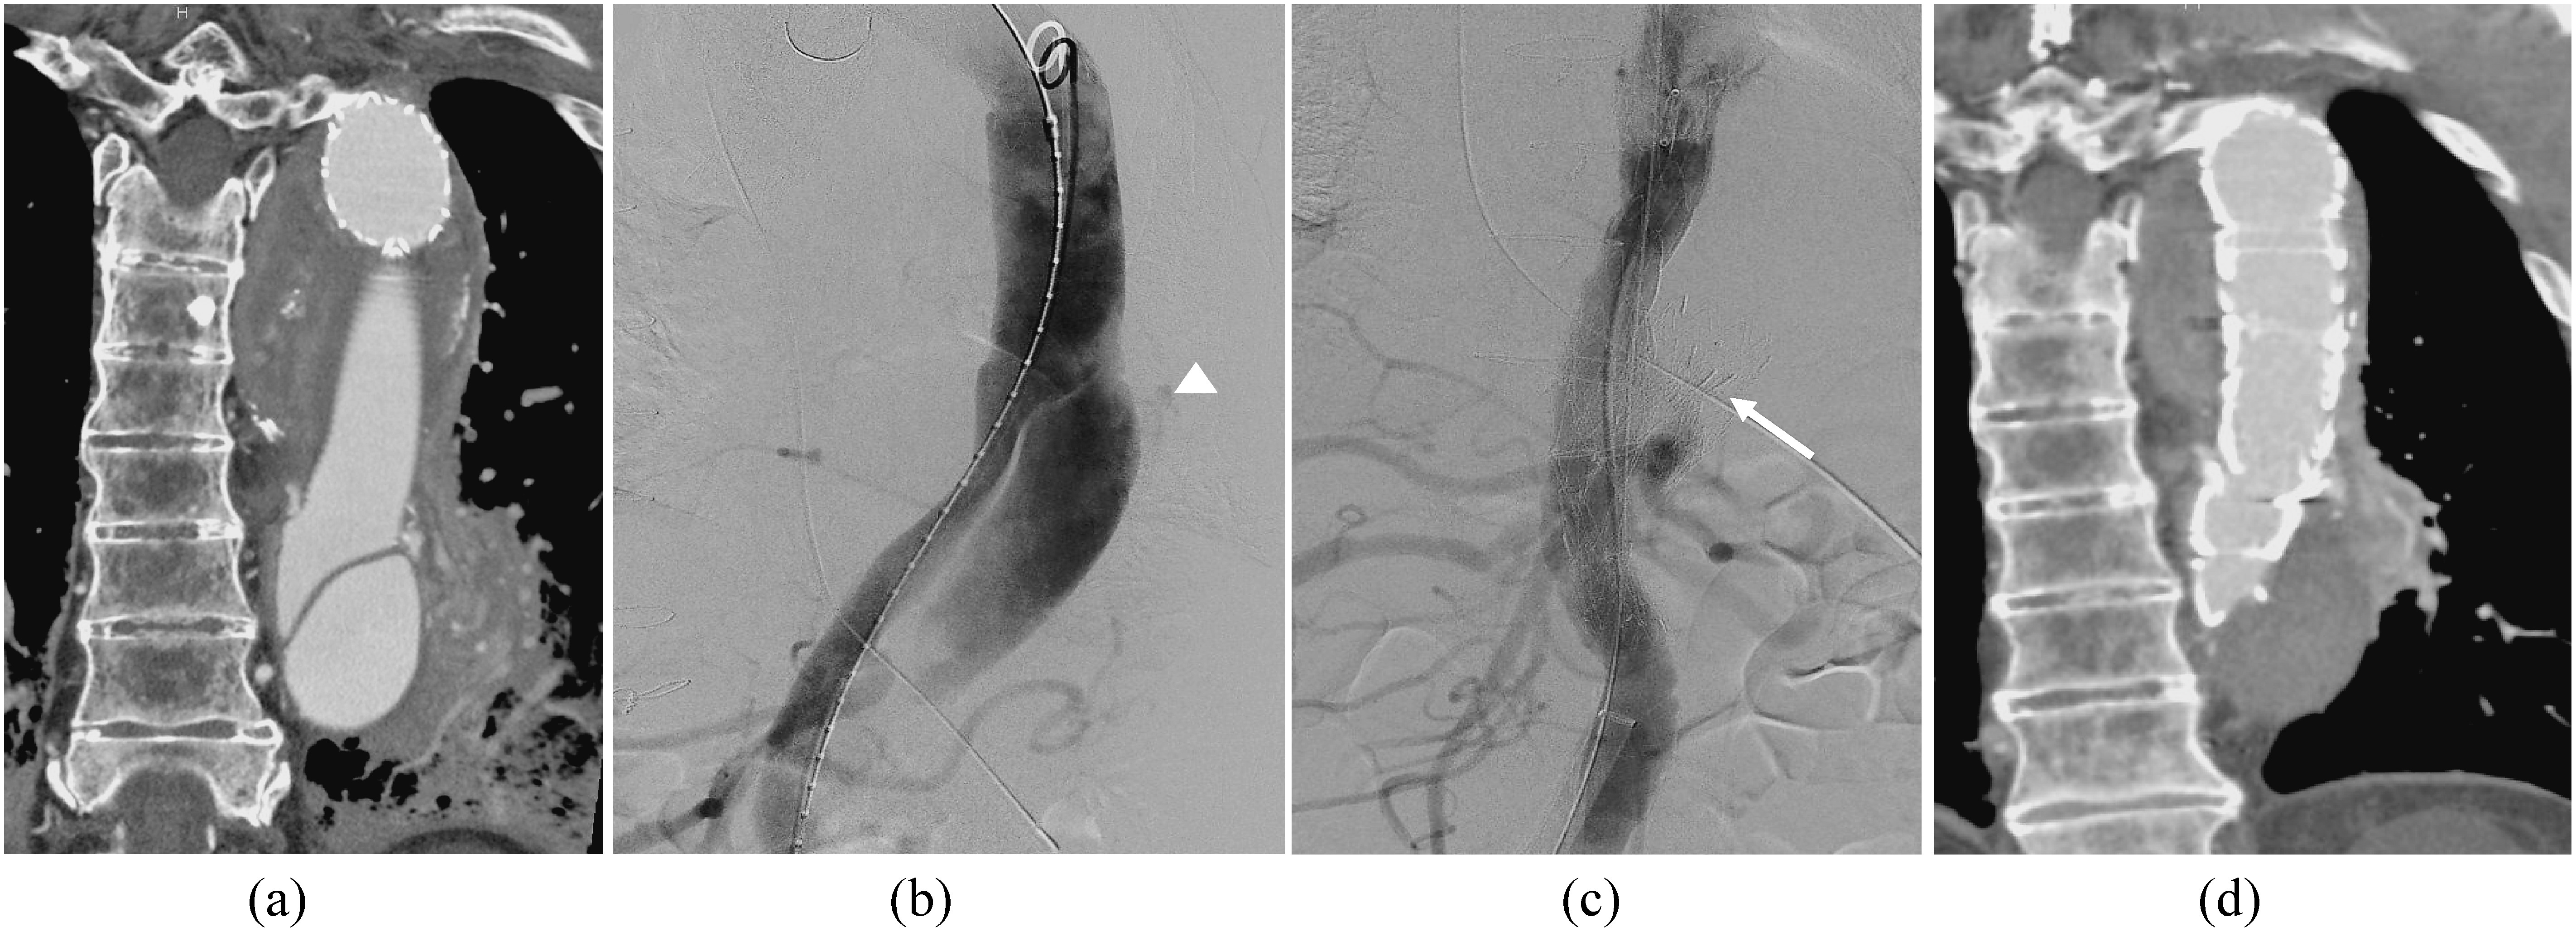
Fig. 6 A man in his 70s with hemoptysis after type A aortic dissection treated by total arch and descending aorta replacement and TEVAR. (a) Preoperative CECT shows hematoma around the anastomosis. (b) Aortography shows minor extravasation (arrowhead) close to the anastomosis and multiple distal entry points. (c) Postoperative TEVAR with the candy-plug technique shows no residual FL flow above the candy plug (arrow) on completion aortography. (d) Postoperative CT 10 days later shows FL thrombosis above the candy plug with a reduction in hematoma volume.
